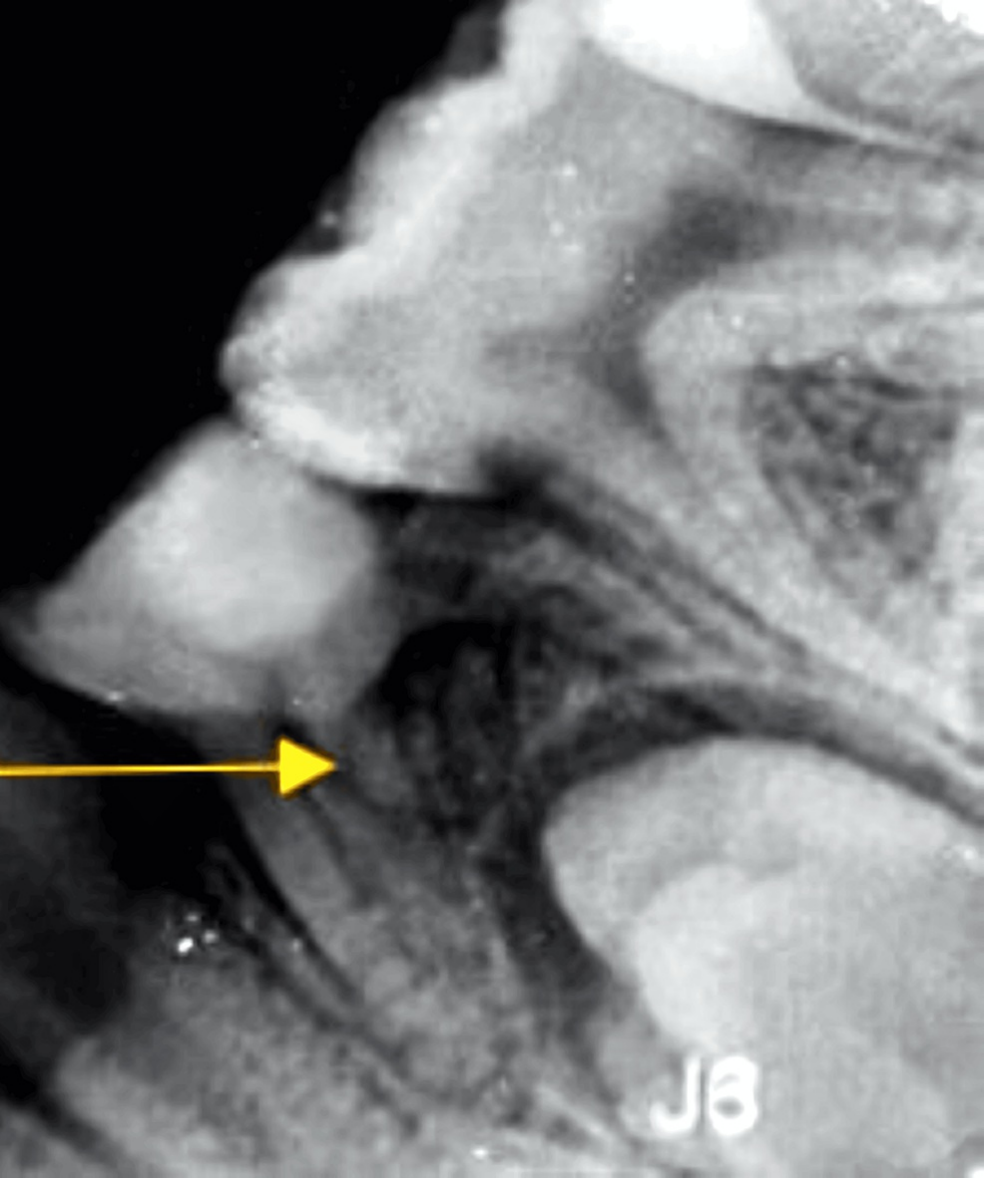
Depiction of the radiograph of RE of right deciduous mandibular first molar at nine-month follow-up RE: Radix entomolaris
